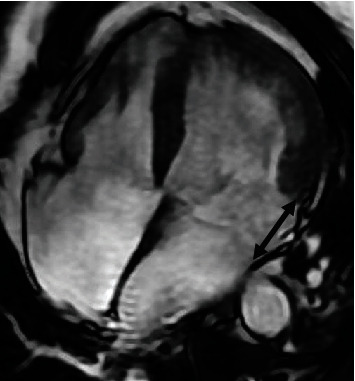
SSFP cardiac MRI image demonstrating a large gap between the insertion point of the posterior mitral leaflet and the top of the lateral wall myocardium—mitral annular disjunction (double-headed arrow).
